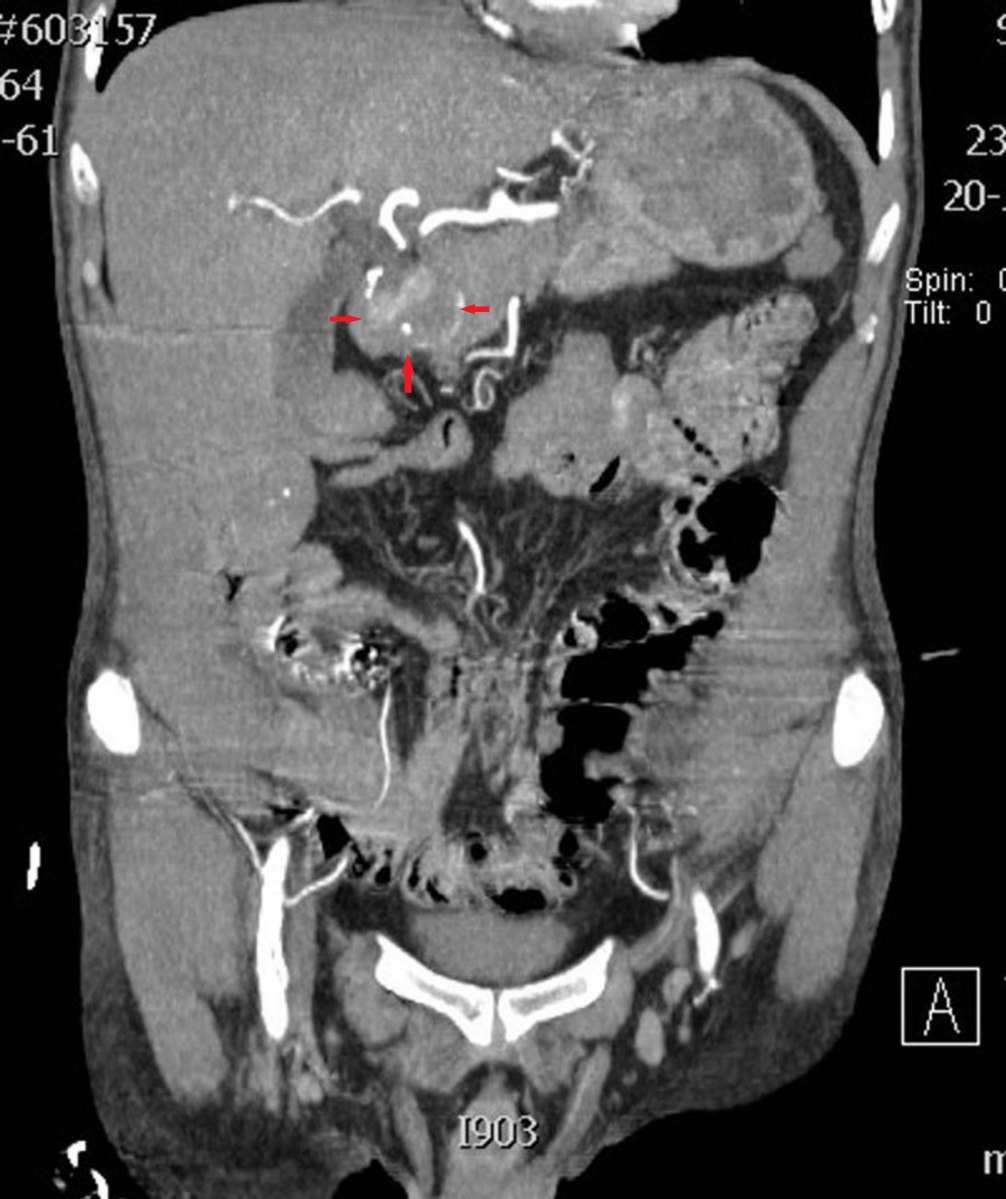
Computed tomography (CT) of the abdomen (axial view) showing blood in the small intestine
